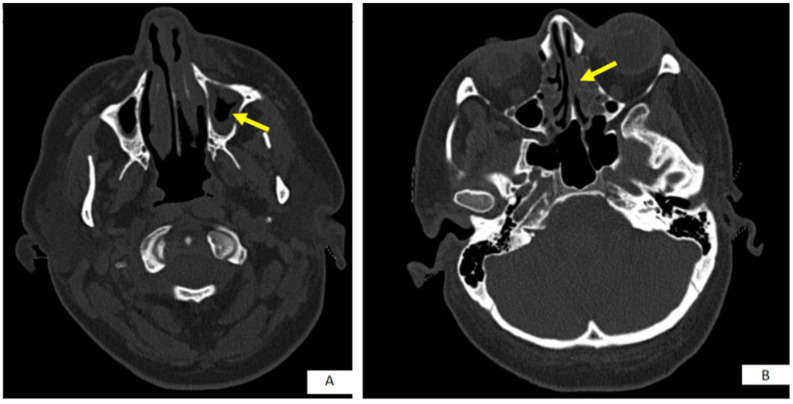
Axial computed tomography of nose and paranasal sinuses shows opacification of (A) the left maxillary sinus (yellow arrow); and (B) the left ethmoidal air cells (yellow arrow).
